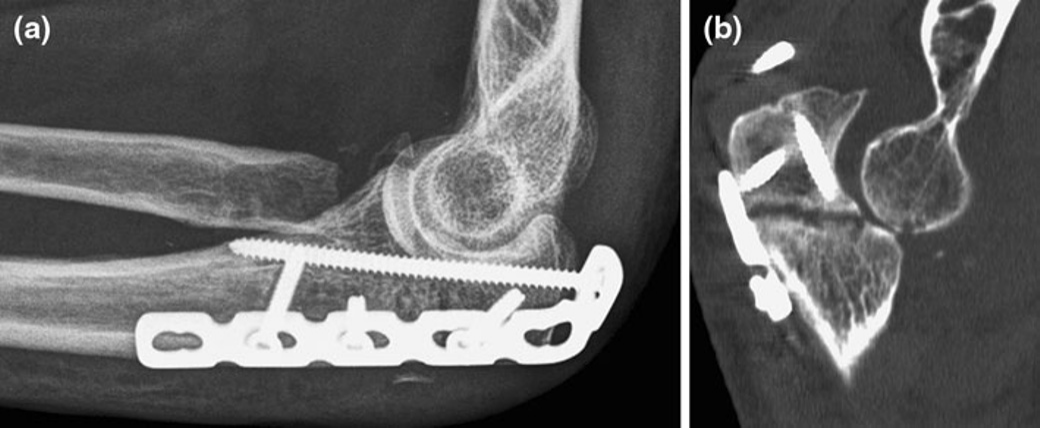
a Plain film could not clearly explain symptoms of pain and limited motion after radial head resection and plating of a Monteggia injury; b The CT-scan provided much more insight showing a nonunion of the proximal ulna and subluxation of the ulno-humeral joint. Case courtesy Peter Kloen (*Source*: Marti and Kloen [26])
